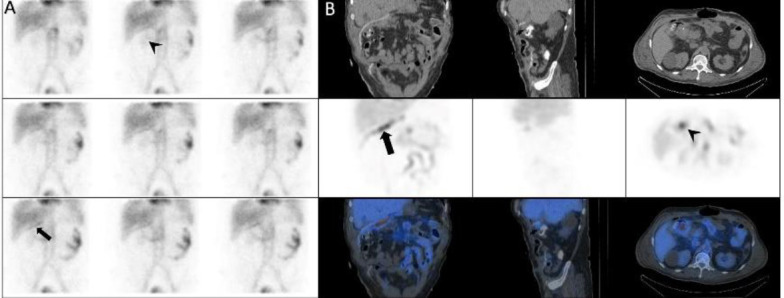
The dynamic (A) and SPECT/CT images (B) showed activity along the lumen of the surgical drain (black arrows). Source of bleeding was duodenal area (arrowhead)
